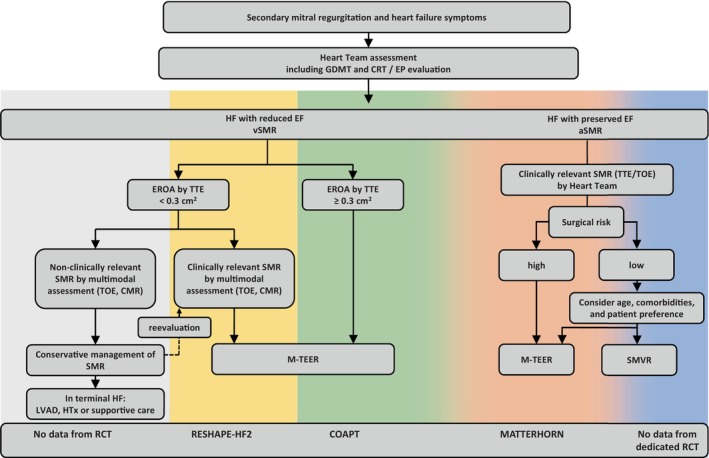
Proposed care pathway for patients with heart failure (HF) and secondary mitral regurgitation (SMR). aSMR, atrial secondary mitral regurgitation; CMR, cardiac magnetic resonance; CRT, cardiac resynchronization therapy; EF, ejection fraction; EP, electrophysiology; EROA, effective regurgitant orifice area; GDMT, guideline‐directed medical therapy; HTx, heart transplantation; LVAD, left ventricular assist device; M‐TEER, mitral valve transcatheter edge‐to‐edge repair; RCT, randomized controlled trial; SMVR, surgical mitral valve replacement; TOE, transoesophageal echocardiography; TTE, transthoracic echocardiography; vSMR, ventricular secondary mitral regurgitation.
